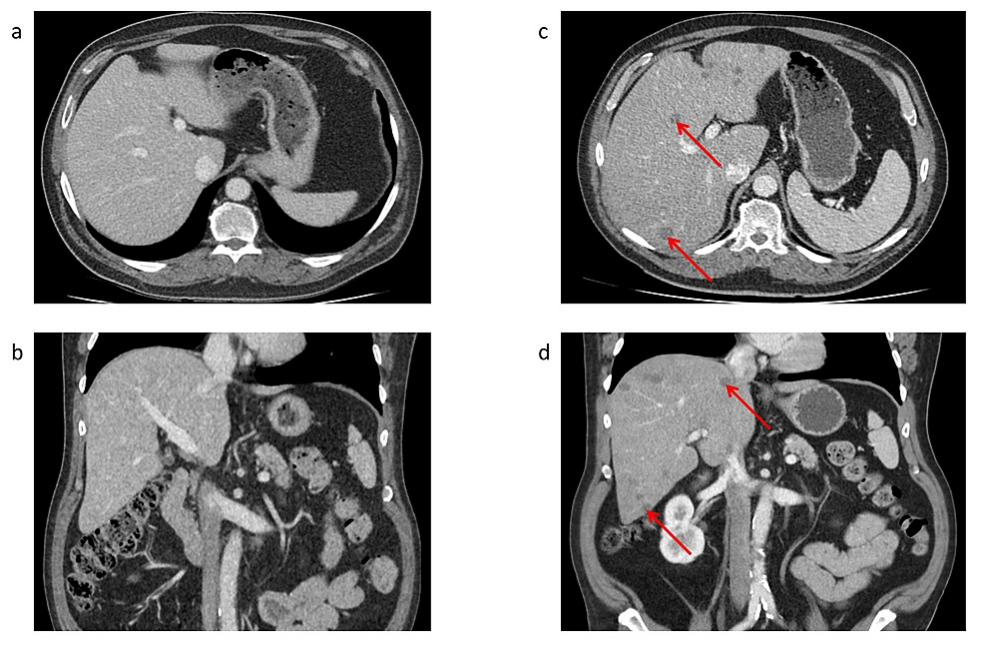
Contrast-enhanced abdominal CT scan Contrast-enhanced abdominal CT scan, (a, b) at time of the diagnosis of autoimmune encephalitis, which reveals normal liver parenchyma, and (c, d) under therapy with glucocorticoids, which reveals multiple hypodense lesions of the liver (arrows). Abbreviations: CT = computer tomography
